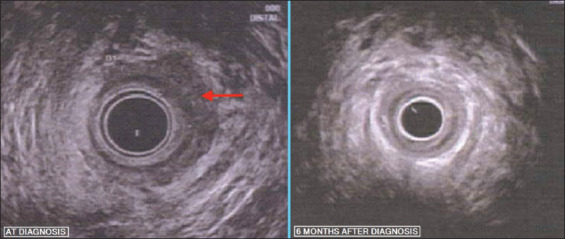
Endoscopic ultrasound at diagnosis and after chemoradiotherapy. The red arrow points toward an anal tumor that was noted on the initial examination.
